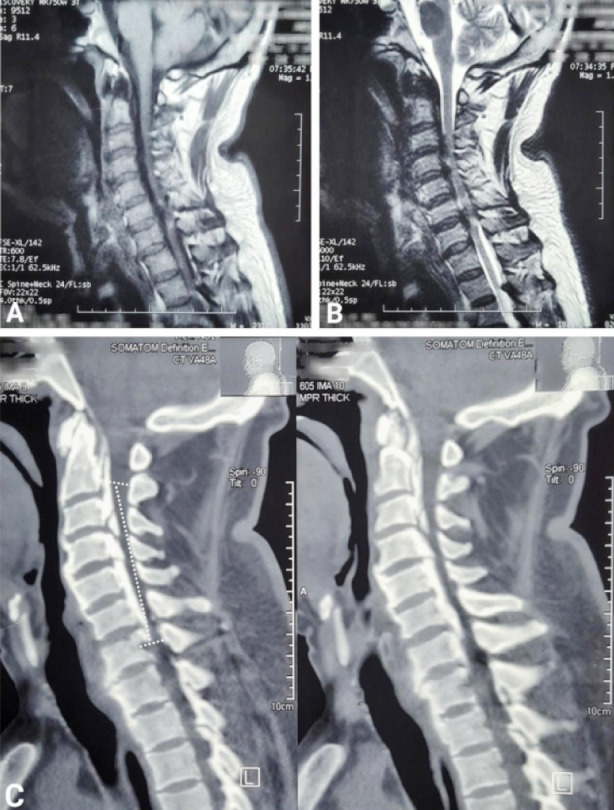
A: MRI cervical spine sagittal T1WI showing hypointense lesion in PLL region from C2 to C7, B: MRI cervical spine sagittal T2WI showing similar lesion from C2 to C7, C: CT cervical spine sagittal view showing mixed type of OPLL from C2 to C7.
